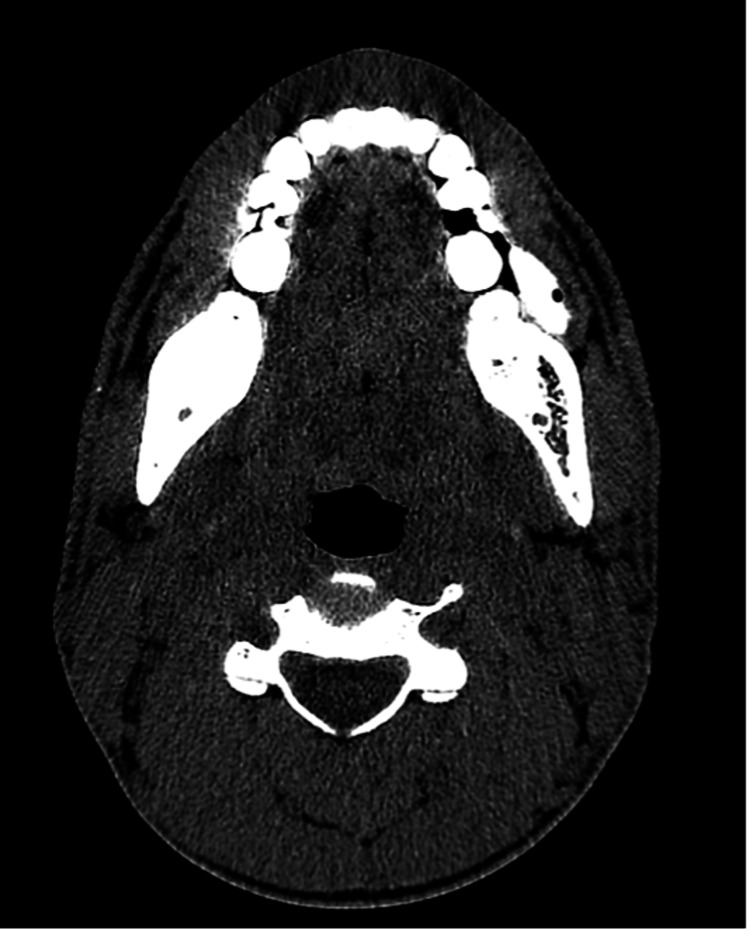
Axial CT non-contrast image in the soft tissue window showing a circumscribed, ovoid, hyperdense mass with internal gas locule in the inferior left buccal space adjacent to the left lower molar teeth. CT: computed tomography
